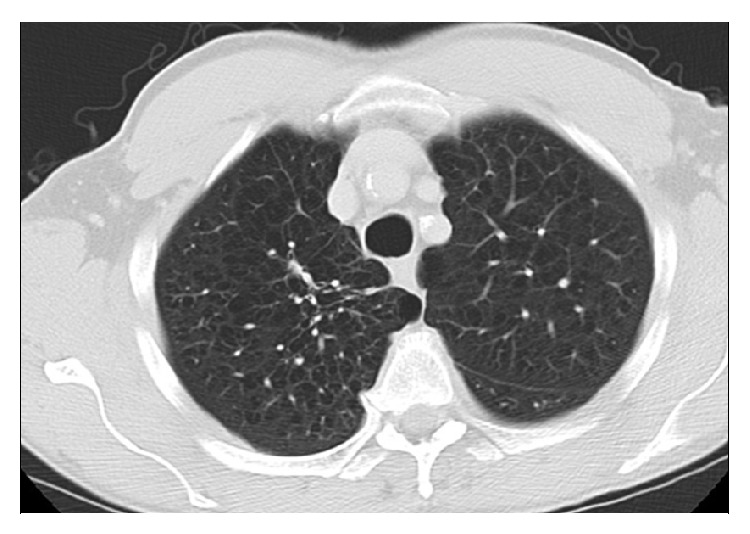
A follow-up CT chest at the end of the triple drug therapy showed a complete resolution of* Mycobacterium kansasii* pulmonary infection.
